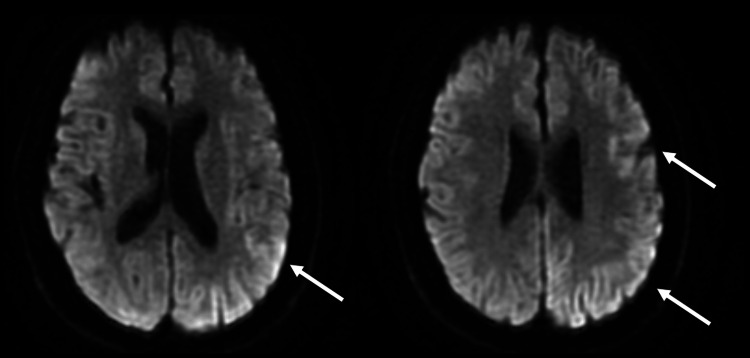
MRI diffusion-weighted images showing a hyperintense signal in the temporo-parietal lobe
